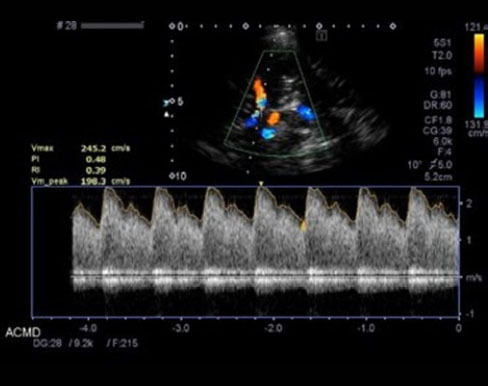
TCD ultrasonography in an adult patient with SCD, demonstrating
